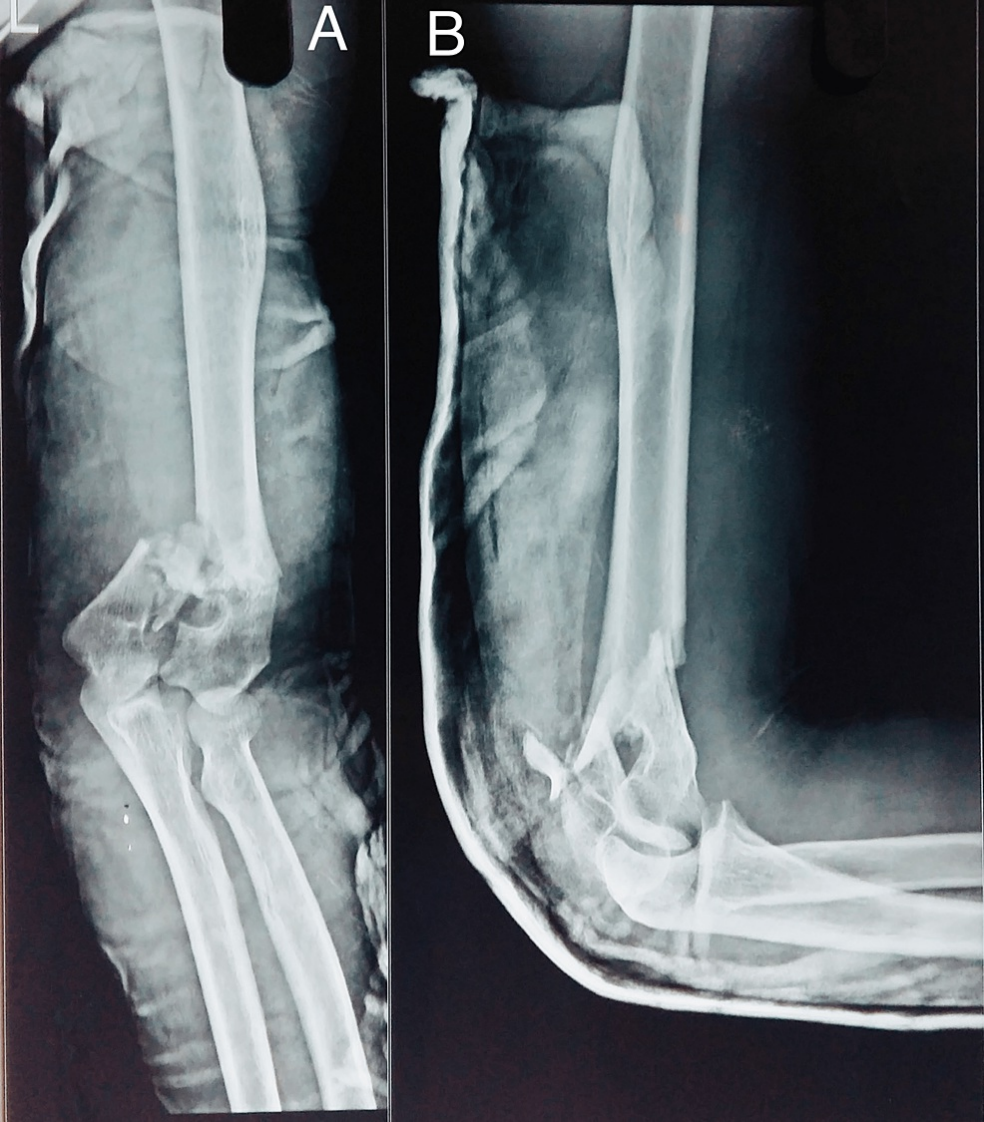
Preoperative radiograph. Preoperative radiograph showing comminuted intra-articular fracture of the distal humerus. A: anteroposterior view; B: lateral view.
